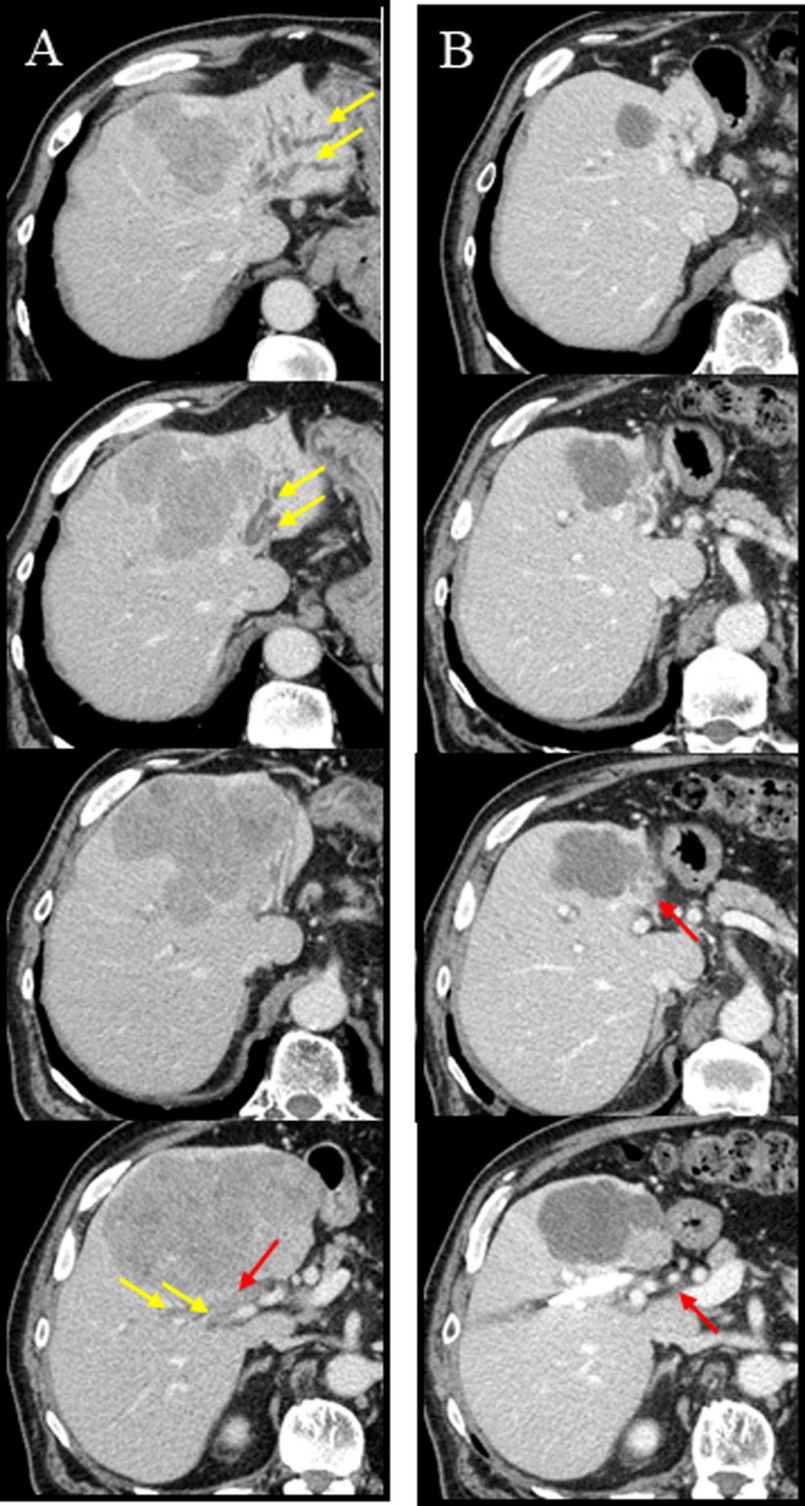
Computed tomography of case 2. A Neoplastic lesion in S4 at the time of diagnosis. Yellow arrows show dilatation of intrahepatic bile duct in anterior, and lateral segment. Red arrows show tumor contact with right hilum region. B Neoplastic lesion in S4 after course of ATEZO/BEV. Tumor contact with hilum region regressed (red allows)
